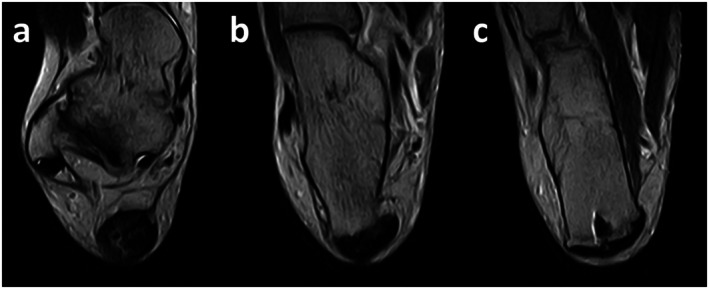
The 5 months MRI shows on axial plane the enlarged remodeled tendon in the most proximal slices (a), which becomes less thick close to the calcaneal insertion (b), especially at the level of more distal and superficial insertion (c).
